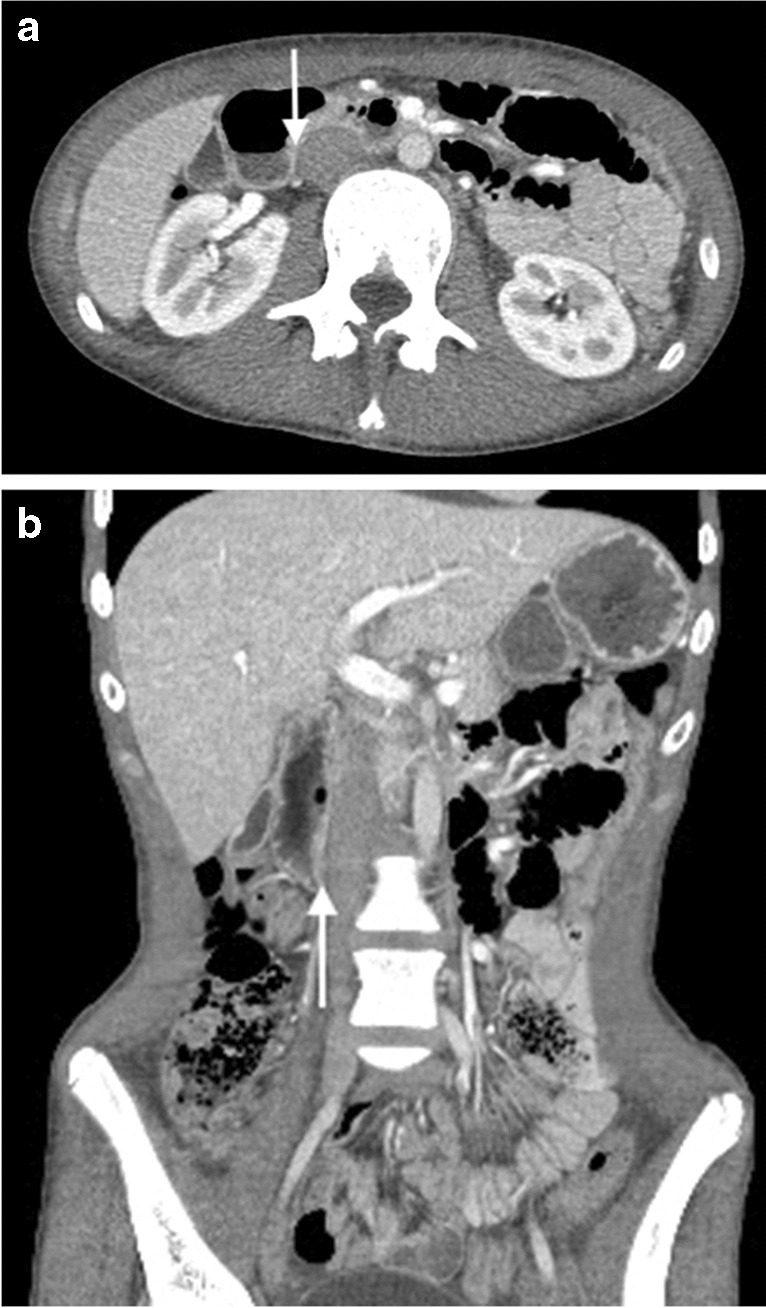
Contrast-enhanced CT images of a 15-year-old female presenting with weight loss, reflux, and dysphagia. a Axial image of the duodenum (*star*) having a posterior relationship to the IVC (*I*) creating the right-of-midline duodenal compression (*arrow*). b Coronal image of the duodenum (*star*) positioned to the right of the IVC (*I*) creating the right-of-midline duodenal impression (*arrow*) on upper GI contrast studies. The patient was diagnosed with an eating disorder and discharged home with support and no diagnosis of superior mesenteric artery syndrome was made
